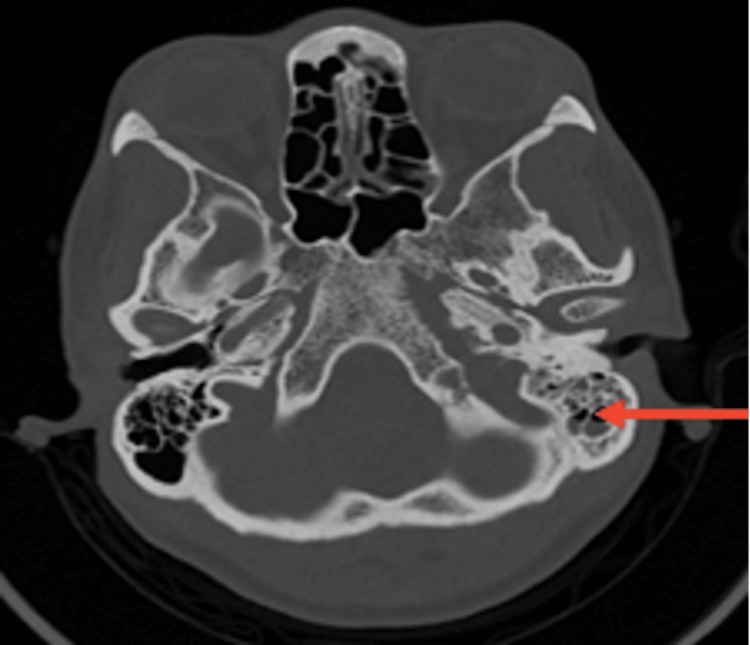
Head CT (axial plane, without contrast) revealing a left-sided otomastoiditis (arrow) CT, computed tomography
